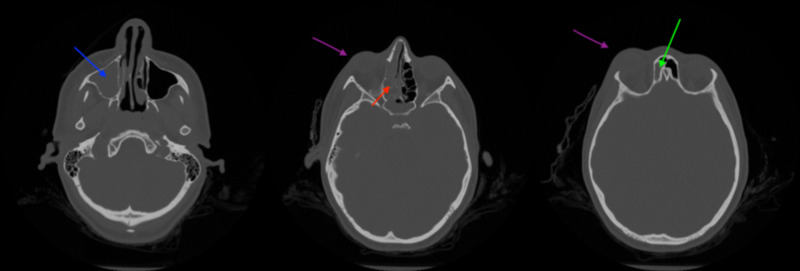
CT without contrast (axial sections) showing right proptosis (purple arrow) as well as complete opacification of the right maxillary sinus (blue arrow), right ethmoid sinus (red arrow), and right frontal sinus (green arrow).
